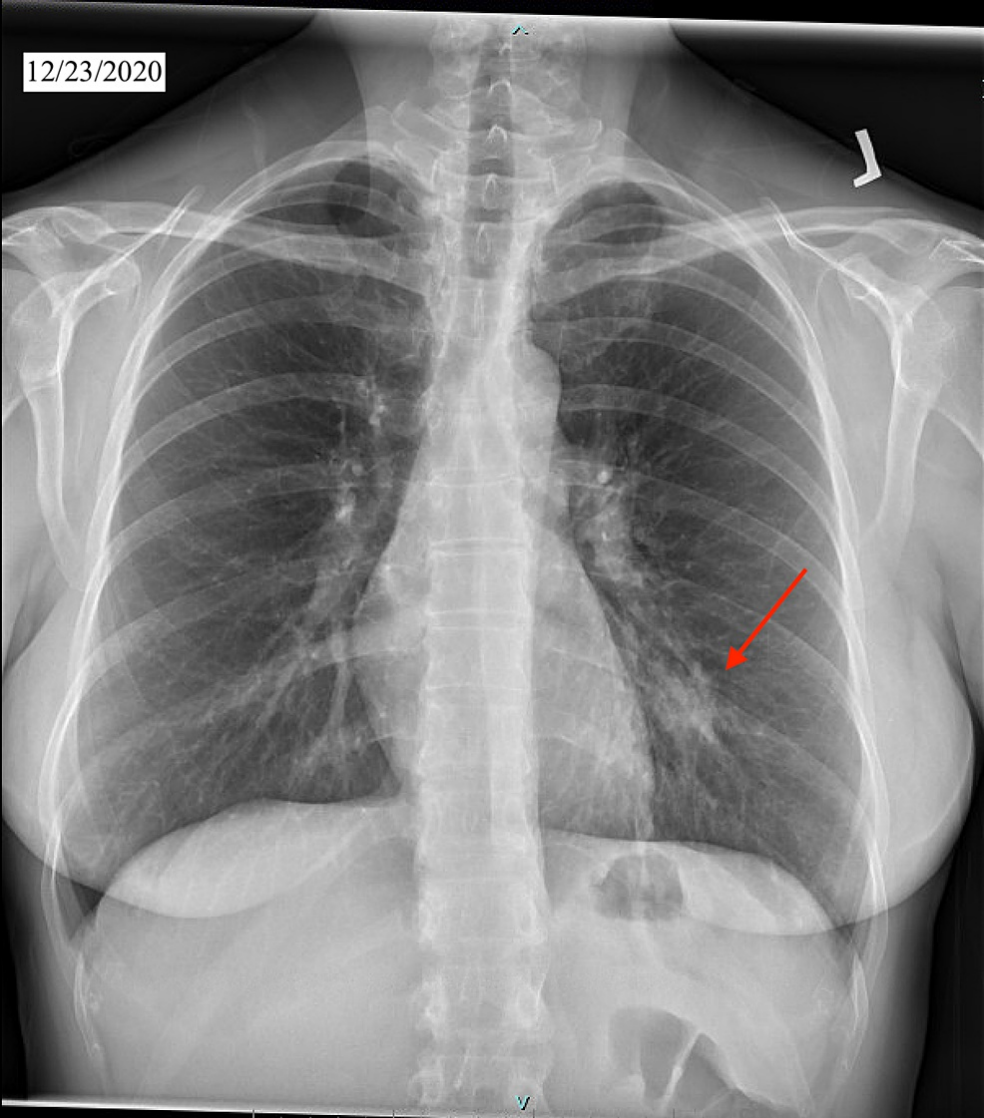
Chest X-ray from 12/23/2020.
